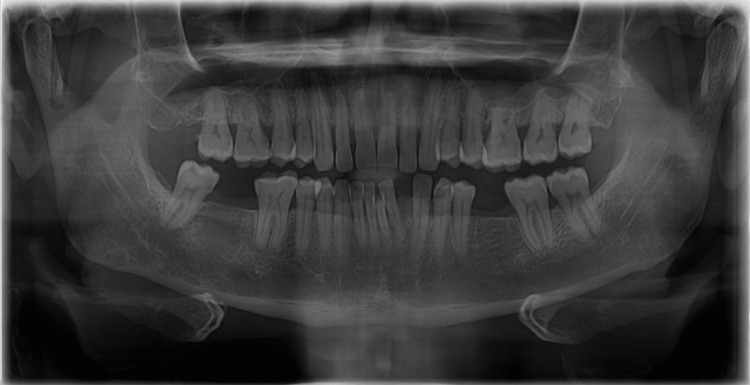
Orthopantomogram showing generalized chronic periodontitis and drifting of 32
